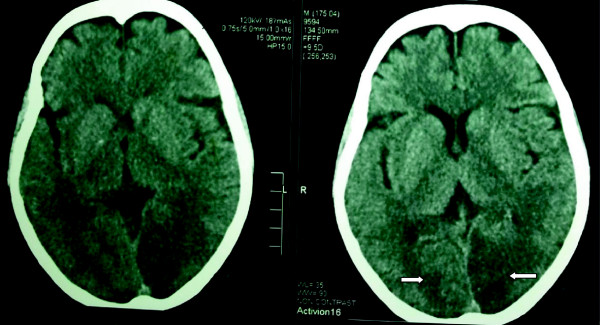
Non-contrast computed tomography of the brain showing bilateral occipital infarcts.
